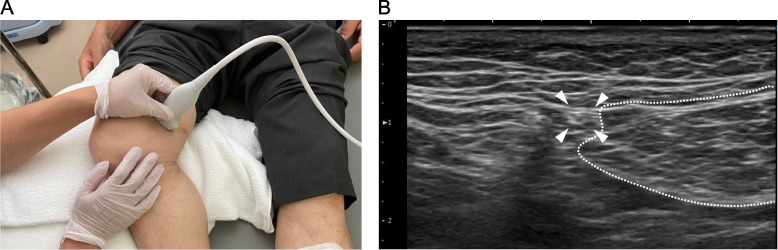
Ultrasound imaging at an especially painful point. A Ultrasound pre-scanning of an especially painful point in the superior medial knee joint region. B Ultrasound scanning reveals a cord-like structure (arrow heads) anterior to the sartorius muscle (surrounded by the white dashed line)
